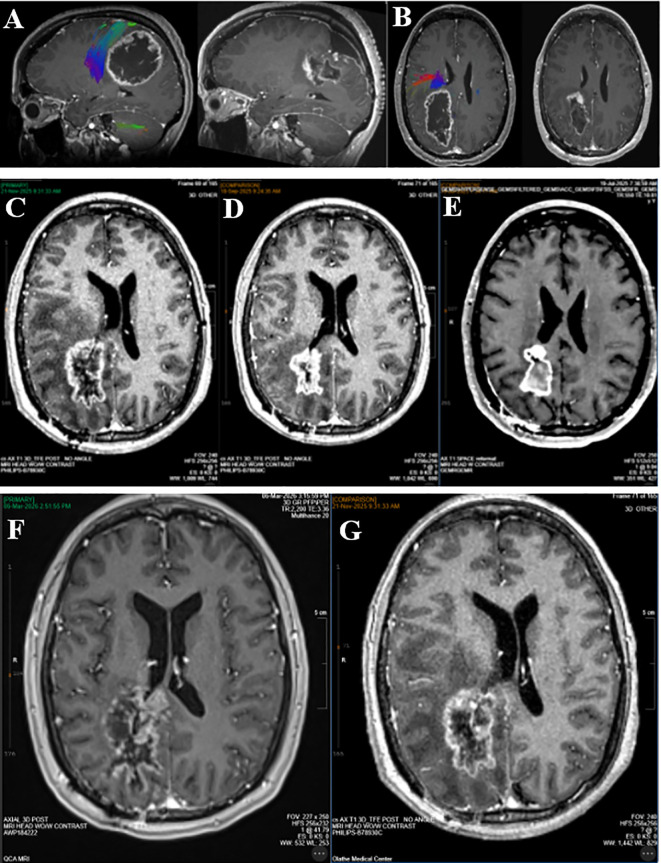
(A) Left, preoperative sagittal T1-weighted MRI with contrast. Corticospinal tracts are indicated in blue (superior-inferior oriented) and yellow (anterior-posterior oriented) with intermediate coloration representing oblique trajectory. Right, postoperative sagittal T1-weighted MRI showed the small residual along the anterior-inferior aspect of the cavity. (B) Left, preoperative axial T1-weighted MRI with contrast. Corticospinal tracts are indicated in blue (superior-inferior oriented) and red (medial-lateral oriented). Right, postoperative axial T1-weighted MRI with contrast showing the small residual along the anterior margin of the cavity. (C, D, E) Various images featuring brain MRI of Glioblastoma patient taken in (C) November 2025, displaying progressive disease, (D) September 2025, taken post-radiation therapy, and (E) July 2025, taken pre-radiation therapy. (F, G) Side-by-side comparison of brain MRI taken (F) March 2026 and (G) November 2025 featuring improvement during Bevacizumab and Repotrectinib combination therapy.
